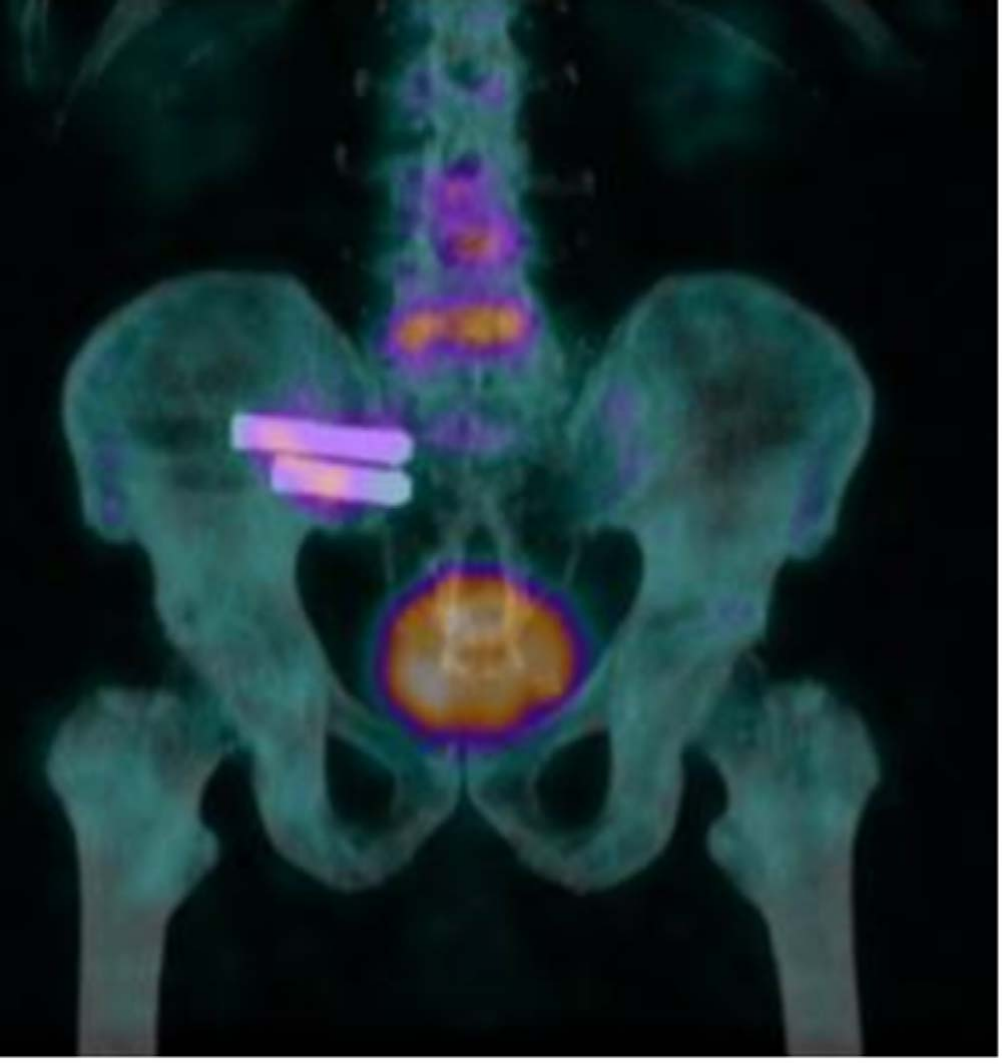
Coronal cut of a SPECT/CT showing cold right SI joint with metalwork insitu and a warm L4–L5 disc space. The patient later underwent L4–L5 fusion.
